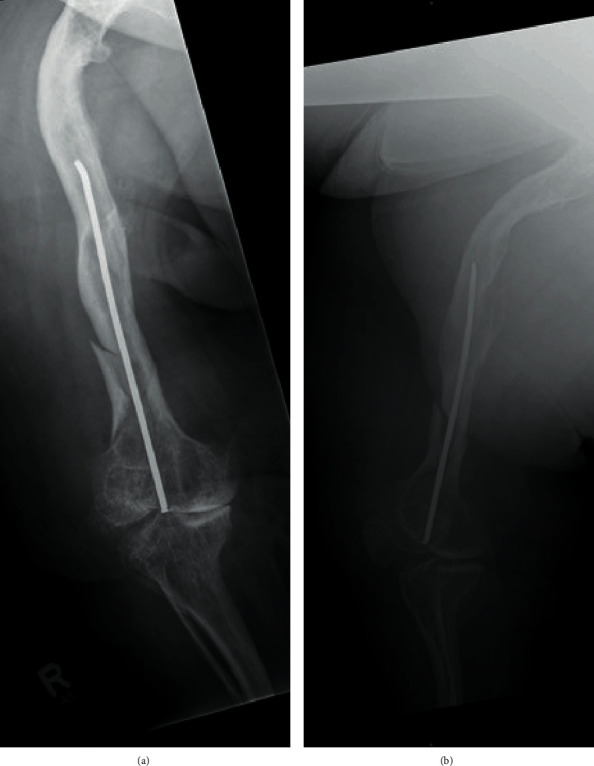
Postoperative radiographs from the second day after surgery. (a) Operated femur in the anteroposterior plane. (b) Corresponding lateral plane. A near-anatomical reduction of the femur has been achieved.
